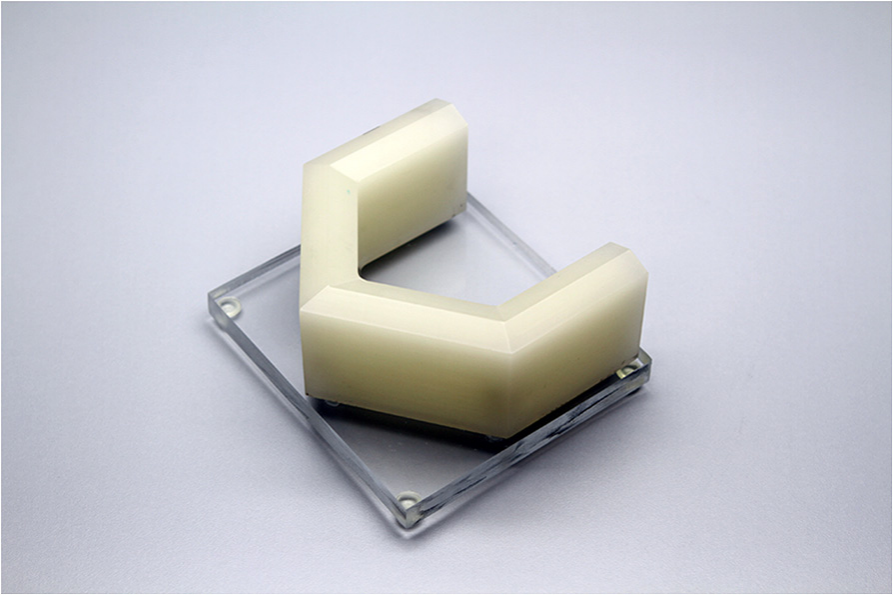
Model and platform design for CBCT and CT scanning.
